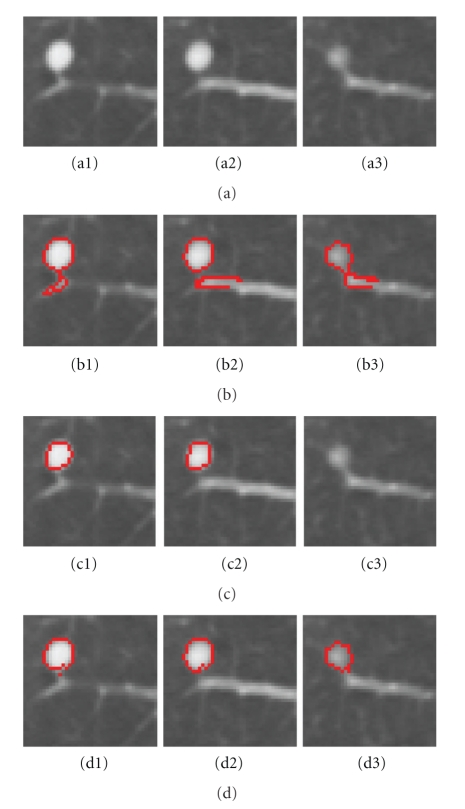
A example of an attached nodule segmentation using different pairwise smoothing energies. (a1–a3) 3D nodule in three contiguous slices in CT; (b1–b3) nodule segmentation using the first term only in (9); (c1–c3) results by using the second term only in (9); (d1–d3) nodule segmentation by using the complete energy defined in (9).
